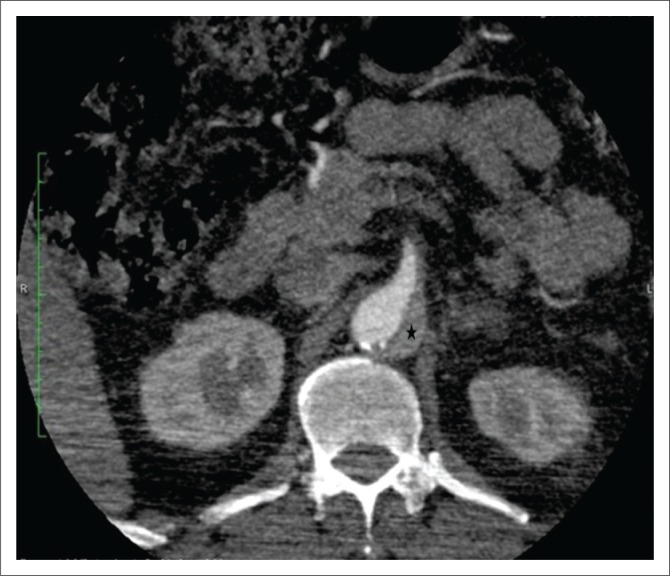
Stanford type B dissection with extension into the superior mesenteric artery. Contrast-enhanced computed tomography showing lesser enhancement in the false lumen (*) as compared to true lumen.
